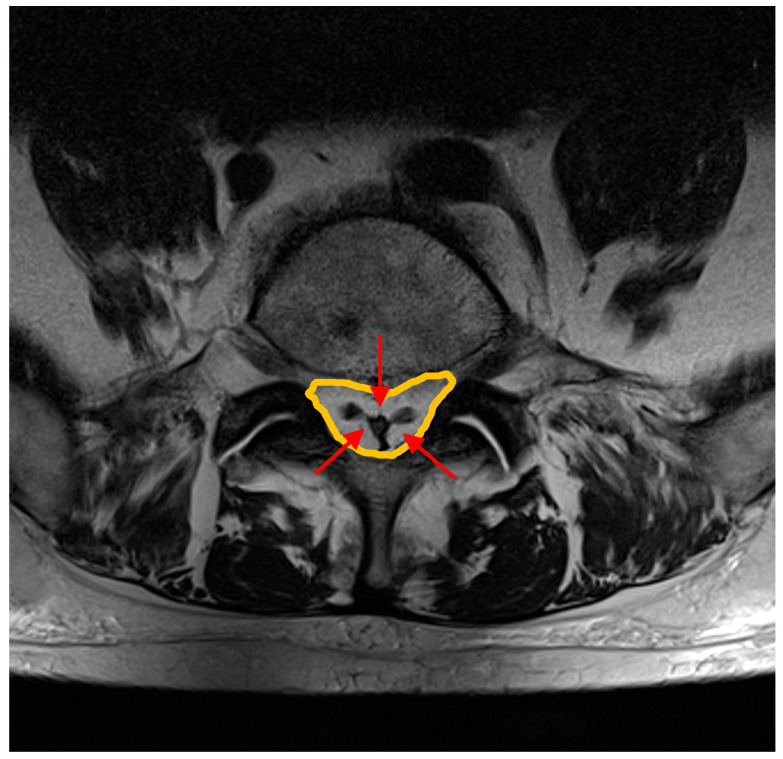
MRI axial T1-weighted sequence shows the presence of excessive accumulation of epidural fat within the spinal epidural space (orange line), resulting in compression of the dural sac and thus leading to a “Y” shaped configuration (indicated by the red arrows); a well-known MRI sign of severe spinal epidural lipomatosis which has implication on systemic health assessment due to the possible linkage with metabolic syndrome. In this case, the patient comes to our attention for low back pain and neurogenic claudication of the lower limbs for about a year, with difficulty climbing the stairs.
